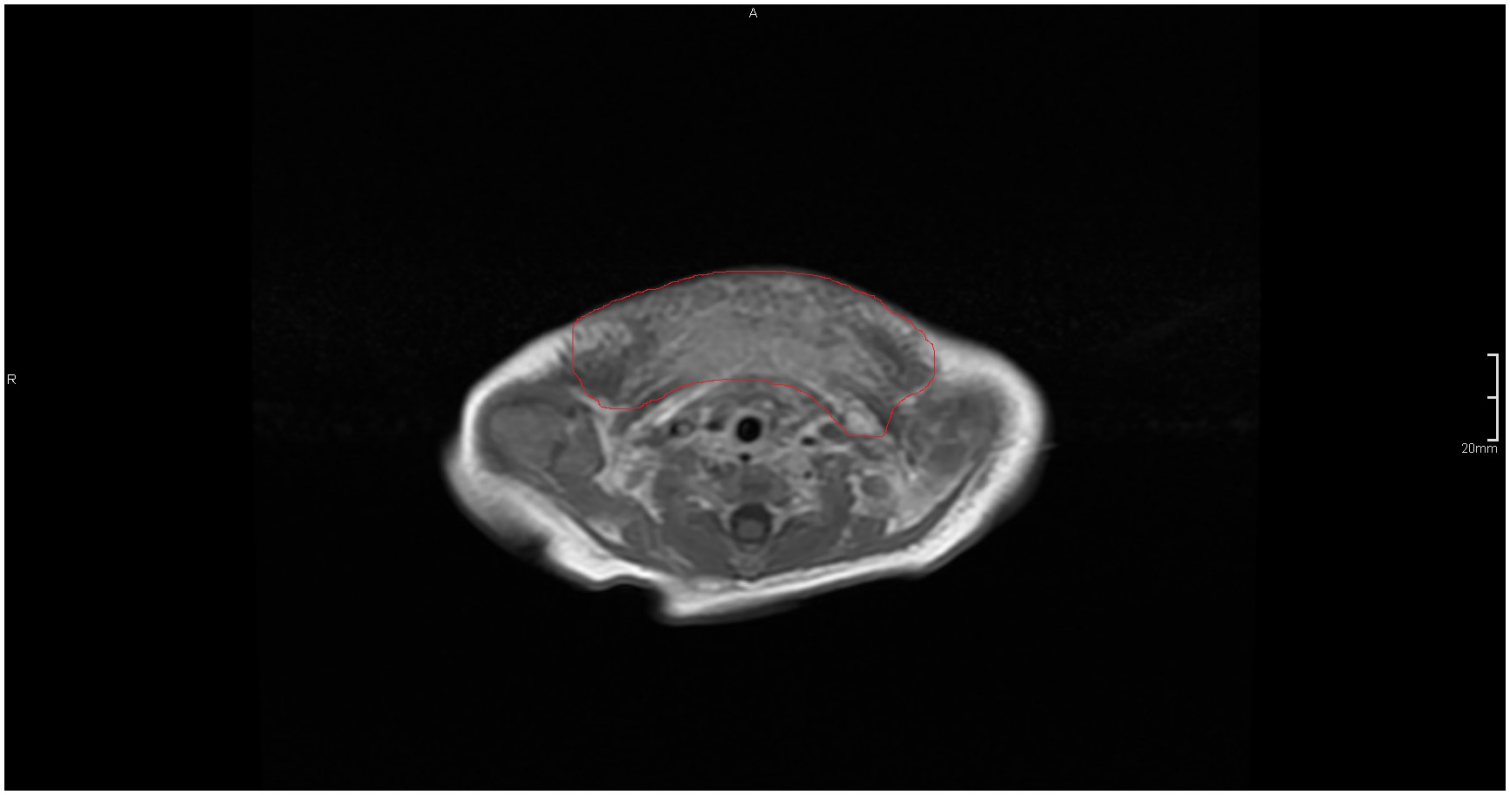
Magnetic resonance imaging: T1 with gadolinium contrast depicts the tumor tissue in white.
